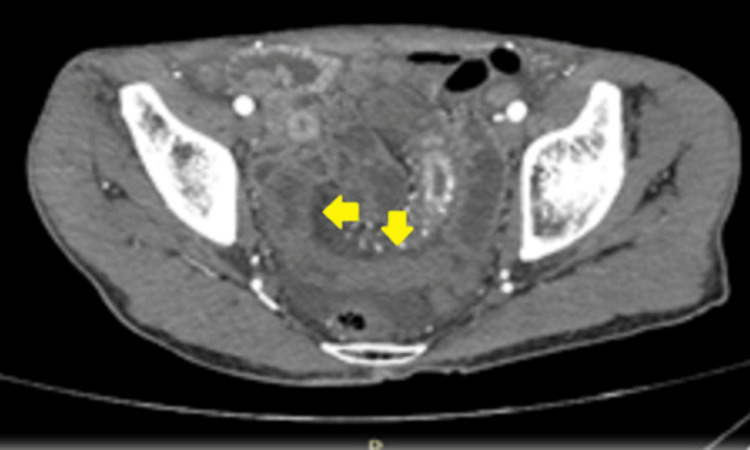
Abdomen and pelvic CT scan with IV contrast Imaging showed a circumferential wall thickening (yellow arrows) in a segment of the distal ileum
